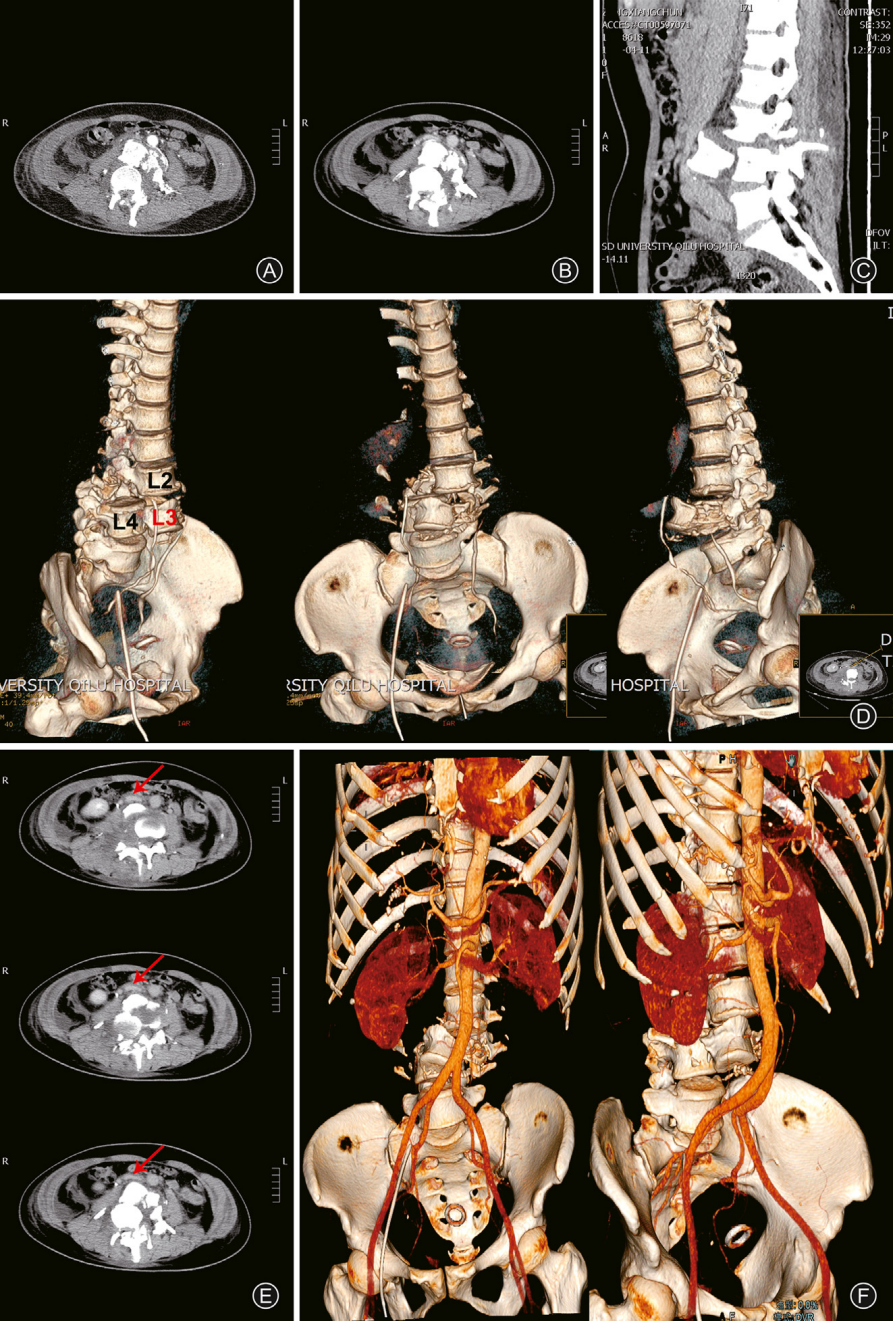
Detailed L_3_ spondyloptosis and its relationship with anterior large vessels. Complete anterior spondyloptosis of L_3_ was confirmed by two separate intensified or non-intensified CT planes (A and B), sagittal scan (C) and reconstruction images (D). Further CT angiography was conducted, compression of large vessels comprising both inferior vena cava and abdominal aorta were discovered from several CT axial planes (E) and reconstruction images (F).
